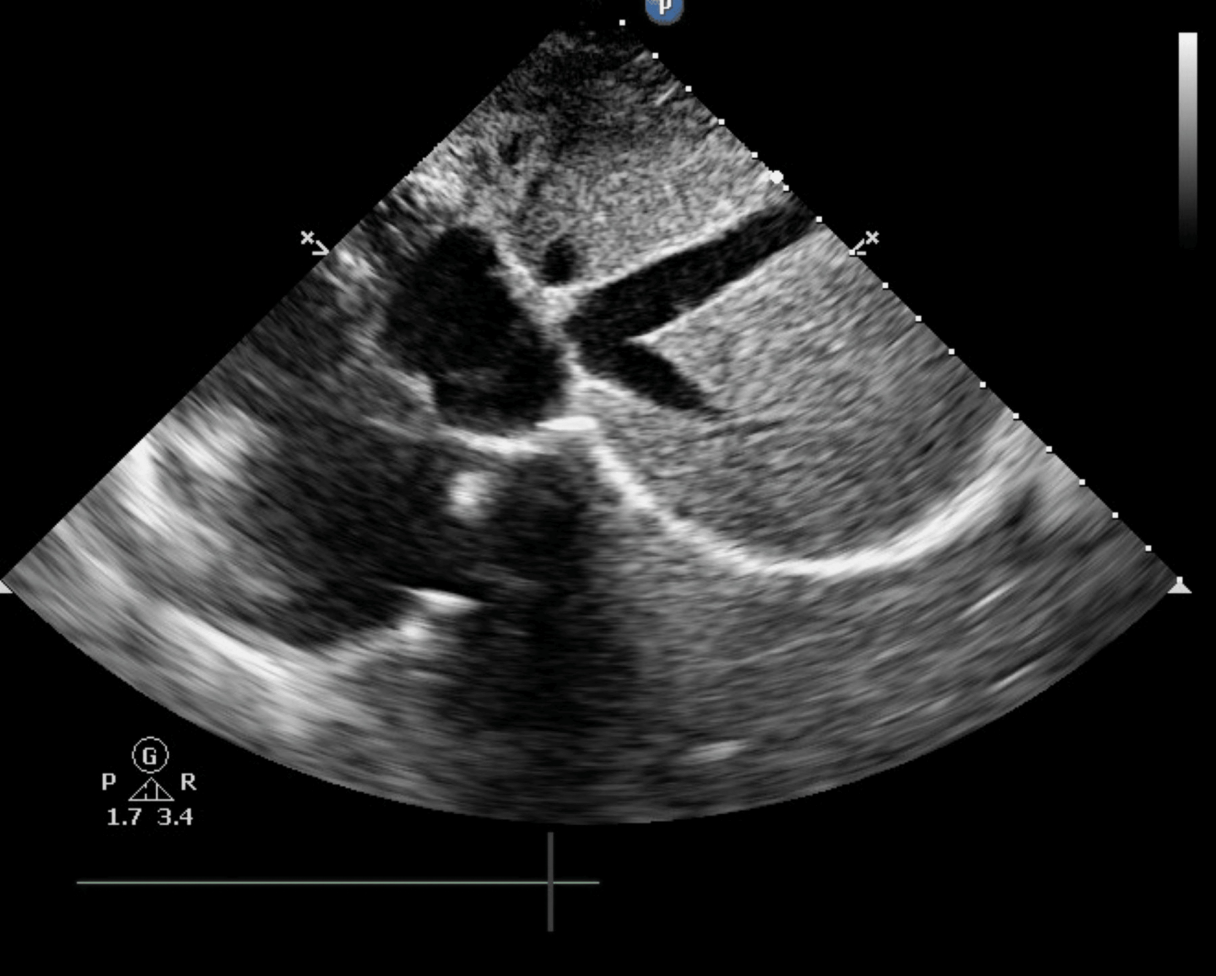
Subcostal TEE view showing the Eustachian valve obstructing the IVC TEE: transesophageal echocardiography, IVC: inferior vena cava
